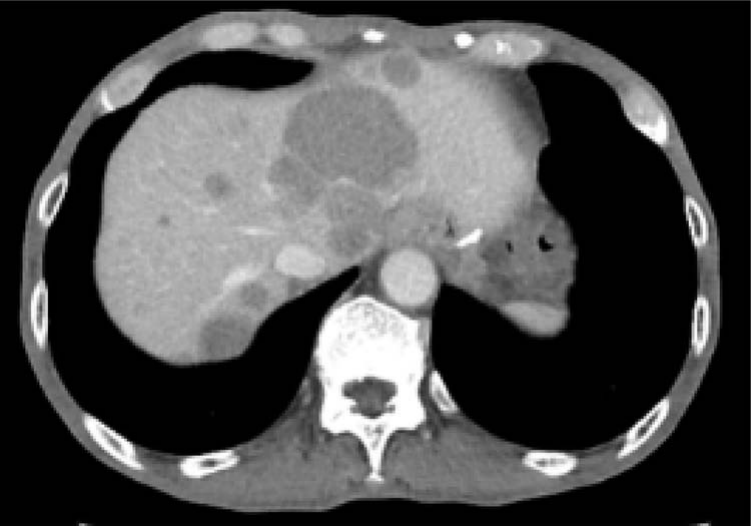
Contrast-enhanced CT showing progression of multiple cystic metastases in the liver.
